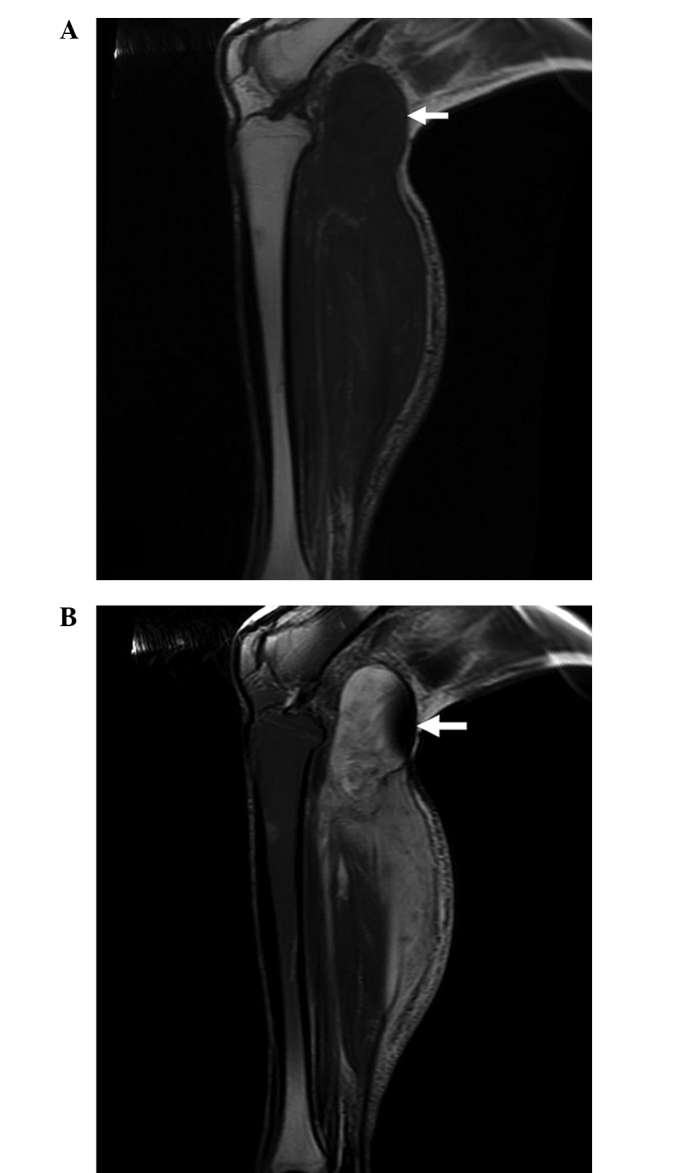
MRI of the sagittal plane of the patient’s right leg showed one large lobulated cystic mass (arrow) filled with debris or tissue thickening, measuring ~5.9×5.4×8.6 cm over the popliteal fossa. (A) T1-weighted MRI showed low signal. (B) T2-weighted MRI showed high signal. MRI, magnetic resonance imaging.
